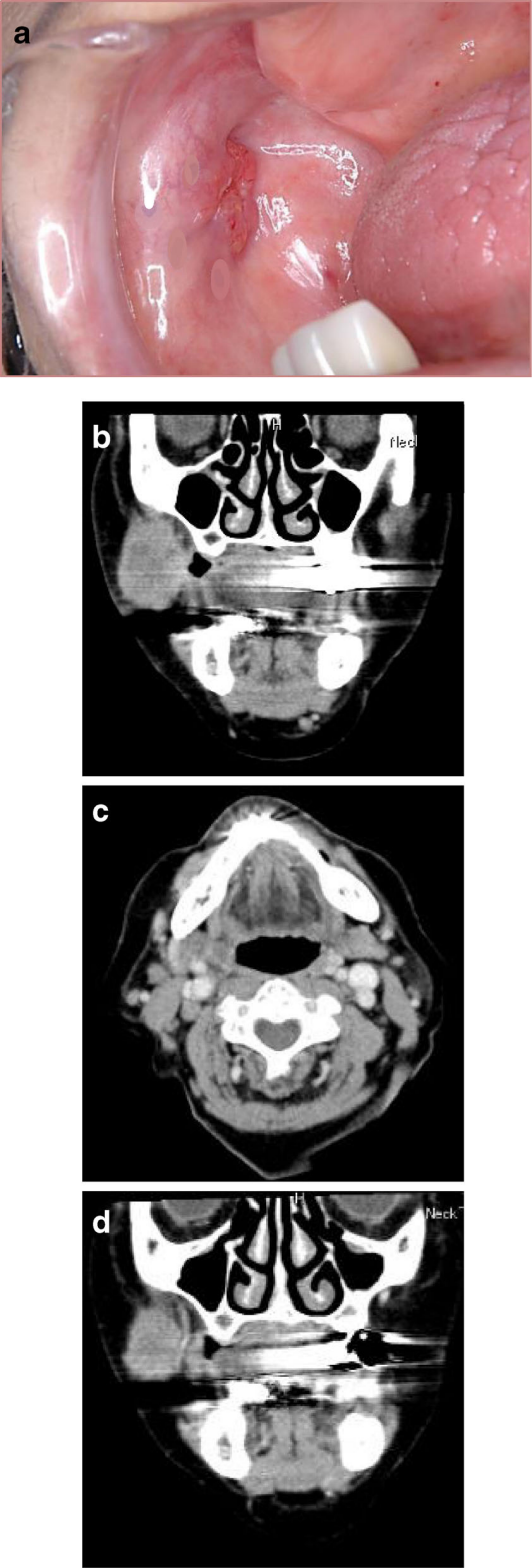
a An intraoral photograph revealing an ulcerated lesion in the right buccal region. b, c Computed tomography demonstrating a homogeneously enhanced lesion in the right buccal region and multiple, enlarged lymph nodes in the right side of the neck. The largest diameter of the tumor on initial assessment (b) and after induction chemotherapy (d) was 34.2 to 26.0 cm, respectively. The tumor exhibited a reduction in size (d) in response to induction chemotherapy using an intra-arterial infusion of high-dose cisplatin and concurrent peroral TS-1
